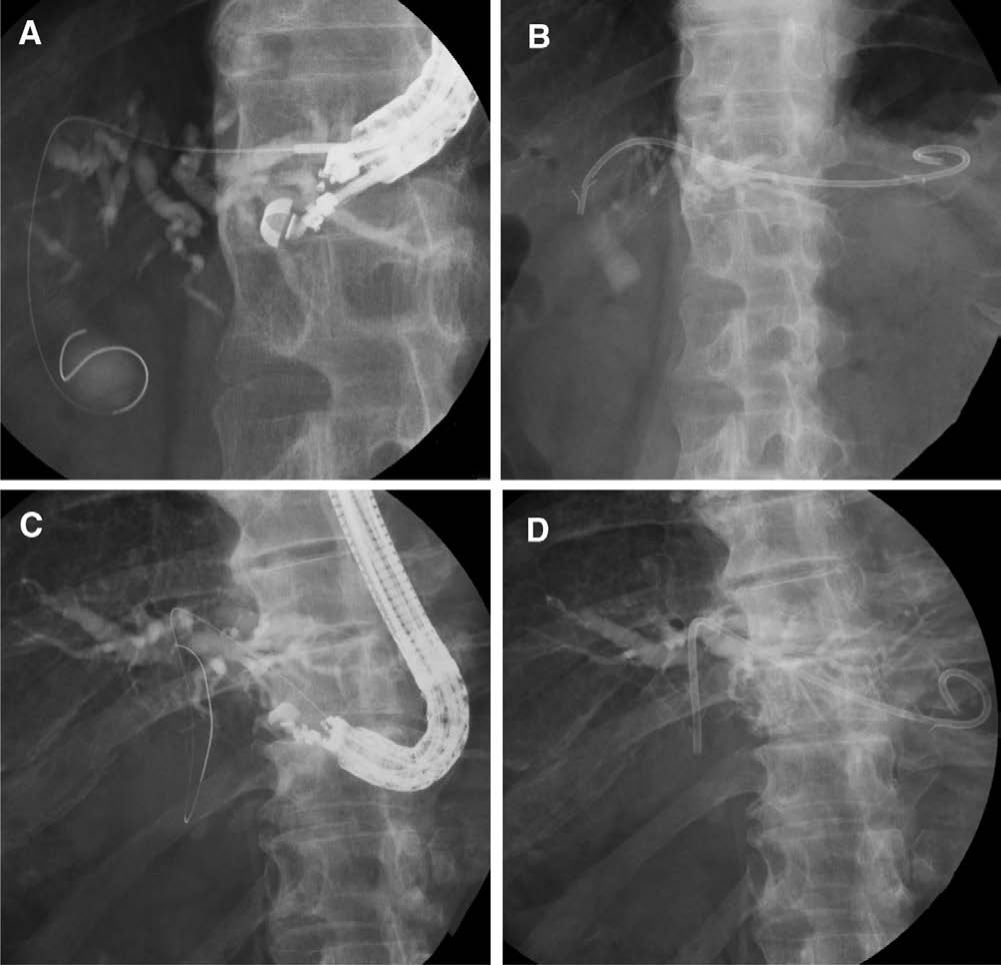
Fluoroscopic images of endoscopic ultrasound-guided hepaticogastrostomy performed using (A and B) a 22-gauge FNA needle combined with a 0.018-inch guidewire and using (C and D) a 19-gauge FNA needle combined with a 0.025-inch guidewire. The visibility of the 0.018-inch guidewire is almost the same as that of the 0.025-inch guidewire. FNA = fine-needle aspiration.
